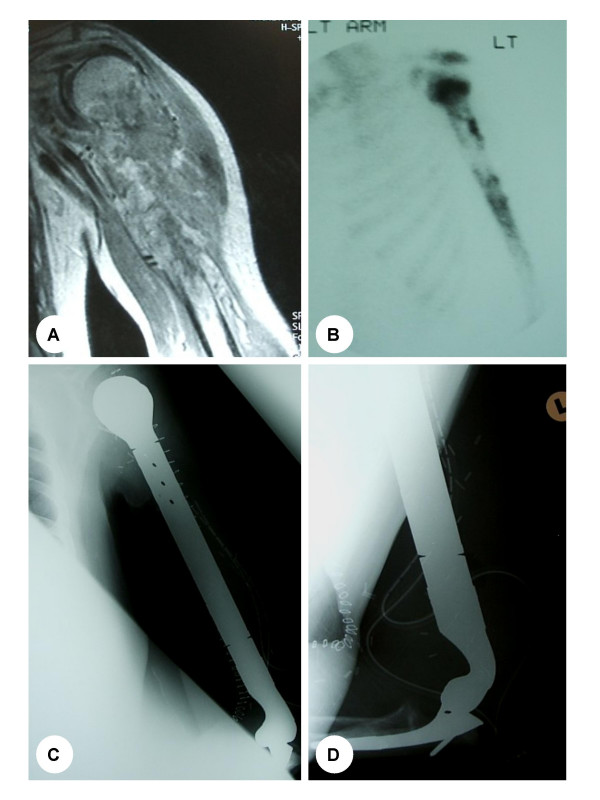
45 year old man with osteosarcoma involving his humerus. (A) Coronal T1-weighted MRI demonstrating the tumour involving the proximal half of the humerus. (B) Bone scan demonstrates increased uptake in the area of the tumour on the delayed static image. (C) and (D) Subsequent operative management comprised of a total humeral resection and endoprosthetic reconstruction.
